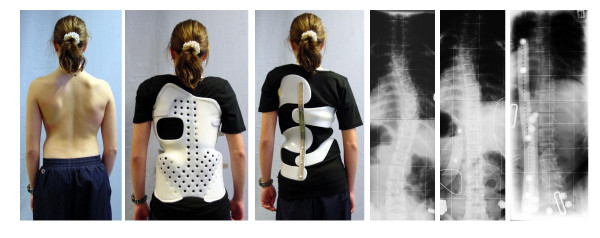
Example of a patient with two different high correction braces. 13-year old girl with AIS (39° thoracic). In the previous brace she had 22° high thoracic, 12° low thoracic and 5° lumbar, while in the Chêneau light^® ^brace she has 22° high thoracic, 8° low thoracic and 11° lumbar. The lumbar correction has not been improved after this x-ray in order to achieve a better balance of curves after treatment and a better cosmetic result. The reduction of material in the Chêneau light^® ^brace compared to the previous brace is clearly visible.
